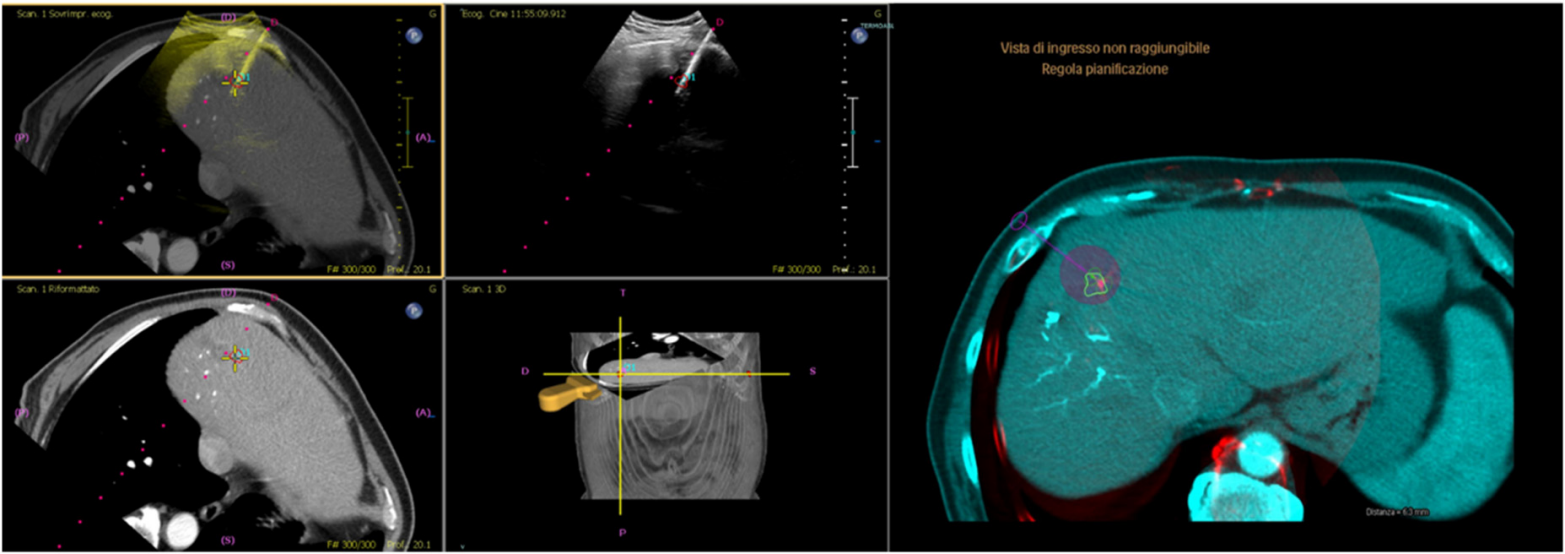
Fusion and CBCT images for a patient with HCC treated with both CBCT fusion and US-CT fusion. Navigation and probe placement were via Percunav Software fusion. After the placement, the correct position is confirmed with CBCT-CT fusion and Volume Ablation Prediction Software was then used (purple) to evaluate and verify adequate margin ablation of the segmented lesion (green). Abbreviations: CBCT, cone beam computed tomography; CT, computed tomography; HCC, hepatocellular carcinoma; US, ultrasound.
